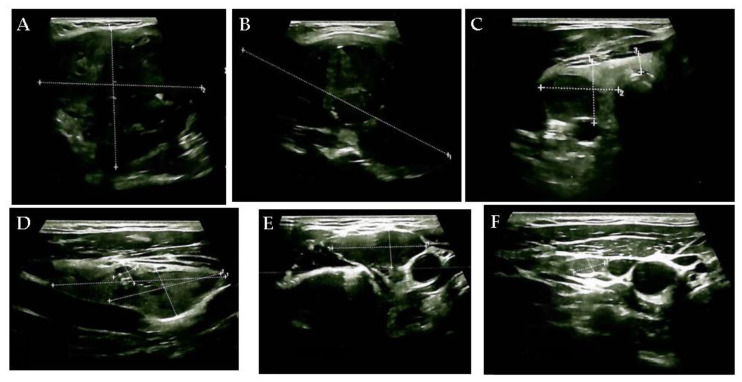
This case study involved a 56-year-old, non-smoking male with a rapidly developing goiter (within 2–3 months) without neck compressive symptoms (the neck mass was detected by self-palpation). His medical history was irrelevant; he was not diagnosed with any endocrine condition, and he was not taking any chronic medication. The patient was living in a non-endemic (non-iodine-deficient) geographic area. A normal biochemical and hormonal baseline panel, including thyroid function and negative thyroid autoimmunity and calcitonin (Table S1), was associated with anterior neck ultrasound features revealing an enlarged, multinodular goiter: the right thyroid lobe (of 2.1 by 2.5 by 5.5 cm) and the left lobe (of 5.75 by 6 by 10 cm) had a hypoechoic, inhomogeneous and multinodular pattern. The left lobe was displaced by a large hypoechoic, inhomogeneous nodular conglomerate of 6 by 8 by 10 cm ((A). transverse plane) with micro-calcifications and macro-calcifications ((B). longitudinal plane), causing tracheal deviation. The right lobe also presented a hypoechoic nodule of 3.7 by 1.6 by 1.9 cm, with increased vascularity and necrosis ((C). transverse plane) and another hypoechoic nodule of 0.75 by 0.7 by 0.7 cm ((D). longitudinal plane). The upper left latero-cervical region showed a hypoechoic, inhomogeneous, highly vascularized lymph node enlargement of 3 by 1.4 cm ((E). transversal plane), and multiple lymph nodes, sized between 0.3 and 1.8 cm. Five other lymph nodes with a hypoechoic aspect, the largest being 0.9 by 0.5 cm in the middle third part ((F) the transverse plane), were found in the right latero-cervical area.
